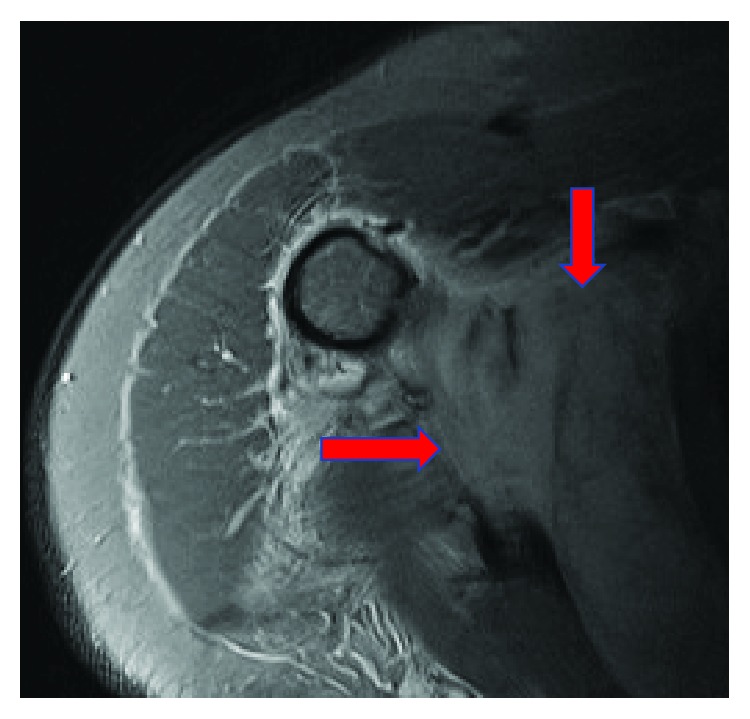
MRI of Rt. shoulder axial STIR sequence demonstrating well-defined ovoid area of hyperintensity.
